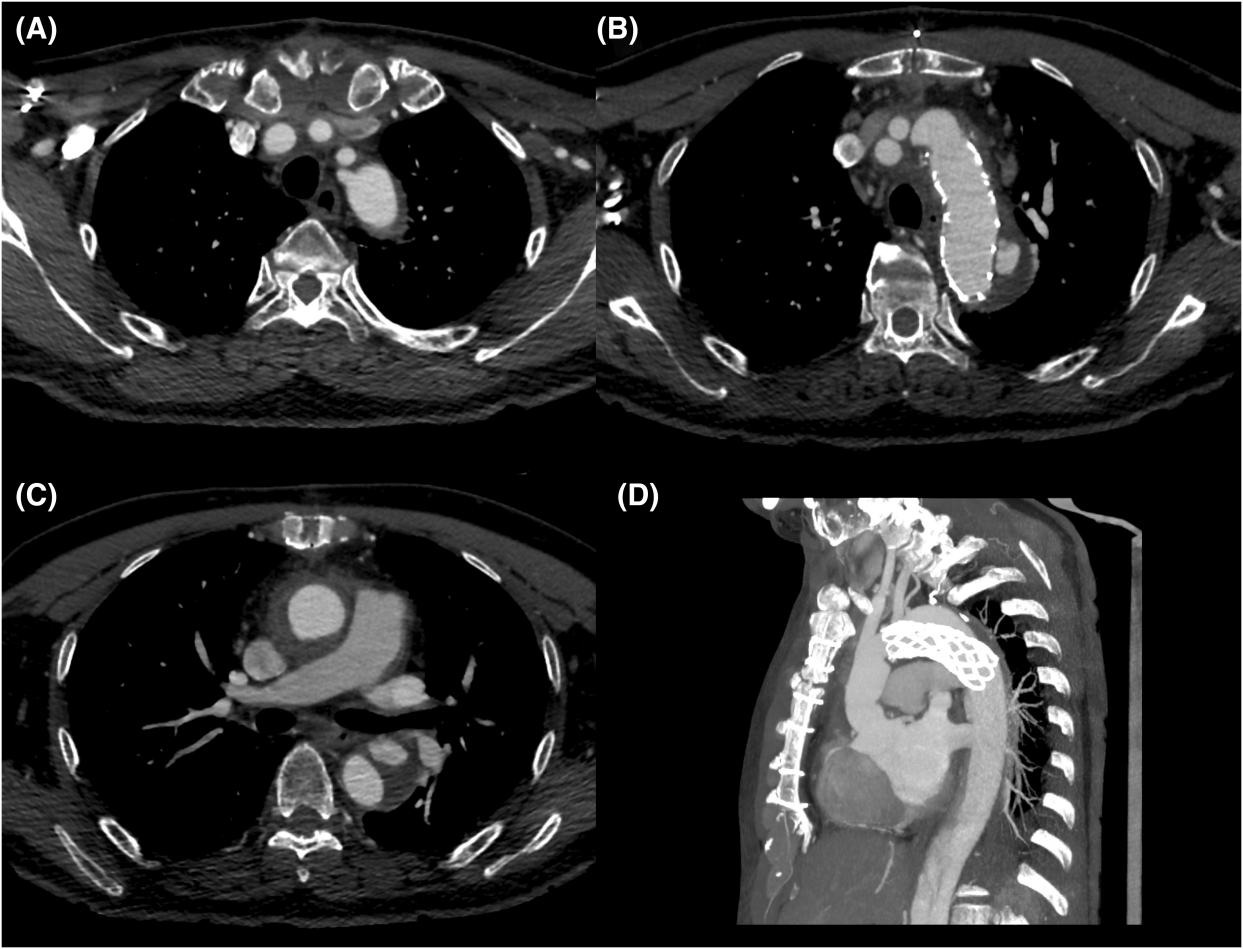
(A,B,C,D) Postoperative computed tomography showing that the antegrade blood flow into the left subclavian artery from the fenestration in the frozen elephant trunk was preserved. Type‐2 endoleak from left vertebral artery to the false lumen in the aortic arch is shown, and endoleak from the fenestration is suspected
